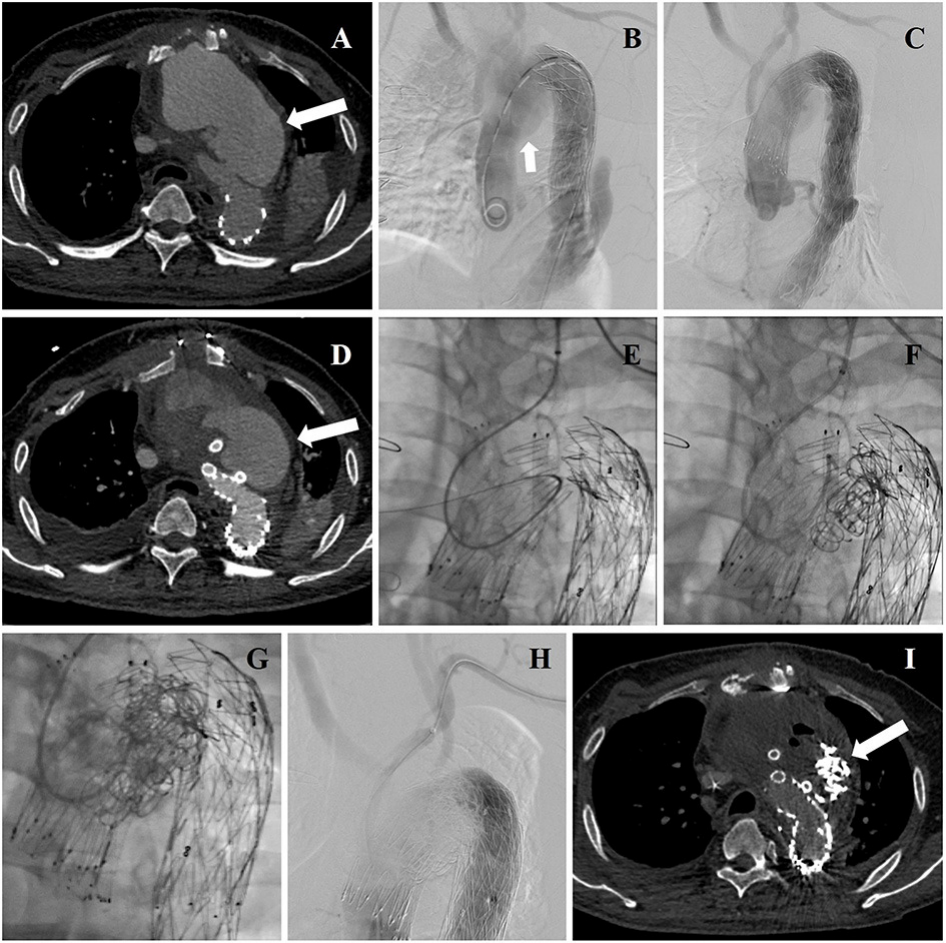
(A) Aortic arch pseudoaneurysm (arrow) was found after TEVAR by CTA admission. (B) Intraoperative DSA identified the location of lesion again (arrow). (C) Intraoperative aortography showing deployment of aortic stent graft and CGs. (D) CTA reexamination revealed post-operative EL-I (arrow). (E) The guidewire and catheter entered into FL through gutters by choosing left brachial artery as the access artery in the second operation. (F) Coils were deployed into FL and gutters. (G) Onyx glues were deployed into FL and gutters through a microcatheter. (H) A final aortography confirmed the patency of arch branches and the exclusion of EL-I. (I) CTA examination was performed 3 months after the second operation showing that endoleak disappeared (arrow).
